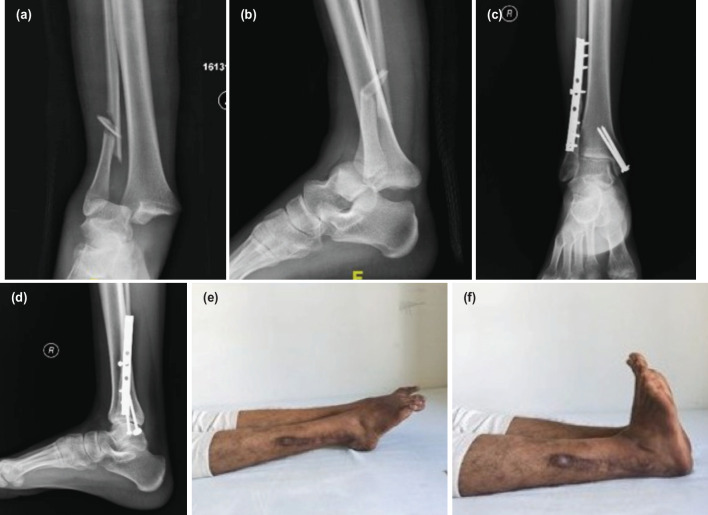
(a, b) Pre-operative radiograph of a 20-year-old male showing ankle fracture dislocation and (c, d) the follow-up radiograph at the end of one year. (e, f) Shows the clinical ankle range of motion at the final follow-up.
